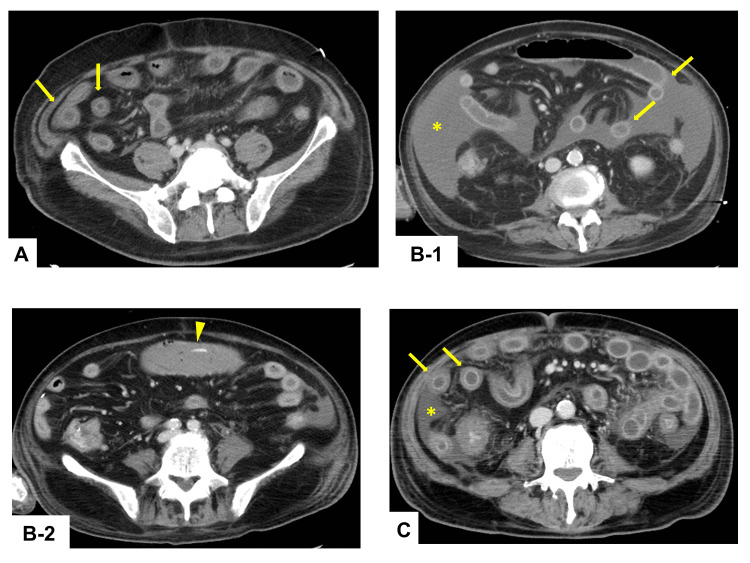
CT findings of each case (A) Patient 1: Day 33. (B-1) Patient 2: Day 51. (B-2) Patient 2: Day 56. (C) Patient 3: Day 32. Axial contrast-enhanced CT images show diffuse bowel wall thickening throughout the small bowel (arrows), along with ascites (asterisks), and small bowel hemorrhage (arrowhead).
